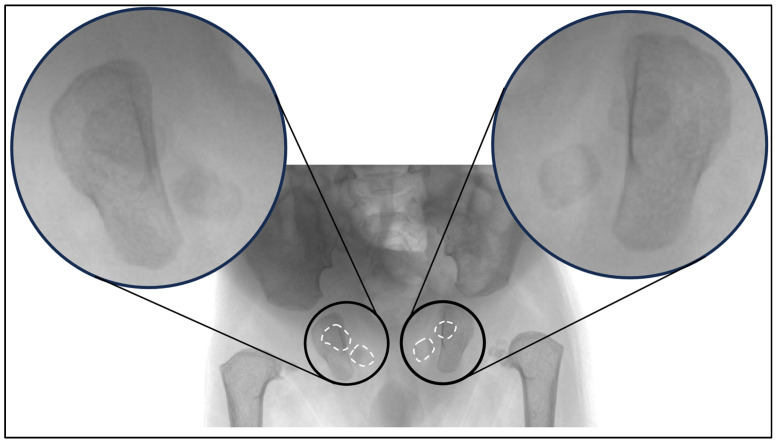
Anteroposterior pelvic radiograph of a 1-year-old child with SEDC showing multiple ossification centers within the superior pubic ramus, visualized as distinct bony parts (white broken lines).
